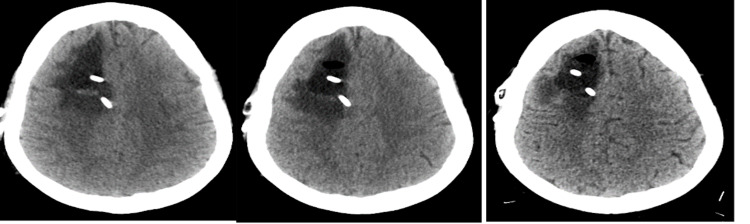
Post-operative CT scans of the head First two on the left are scans done on post-operative day 1 after cystoperitoneal shunt and show the placement of a second right frontal approach ventricular catheter and overall a slight decrease in size. Image on the right end is the CT scan done on post-operative day 4 after cystoperitoneal shunt and shows the unchanged appearance of the right frontal resection cavity with decreasing vasogenic edema.
